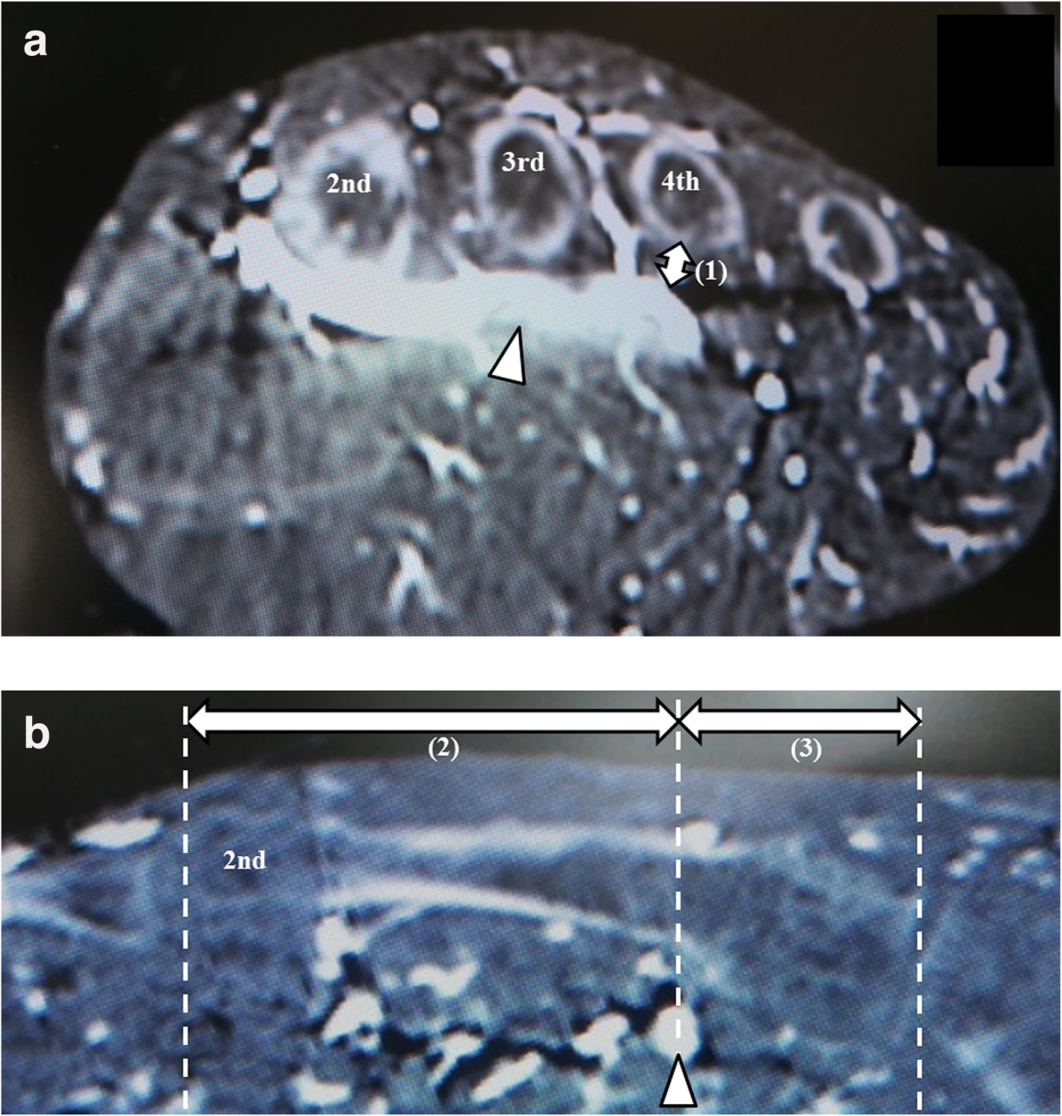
Enhanced CT images showing the following three parameters. (1) The shortest distance from the dorsal aspect of the deep plantar arch to the plantar aspect of the second, third, and fourth metatarsals in the axial plane. (2) The nearest distance from the distal epiphysis to the line passing the deep plantar arch that was perpendicular to the longitudinal axis of the lesser metatarsal in the sagittal plane. (3) The shortest distance from the tarsometatarsal joint to the line passing the deep plantar arch that was perpendicular to the longitudinal axis of the lesser metatarsal in the sagittal plane. The arrow head indicates the deep plantar arch
